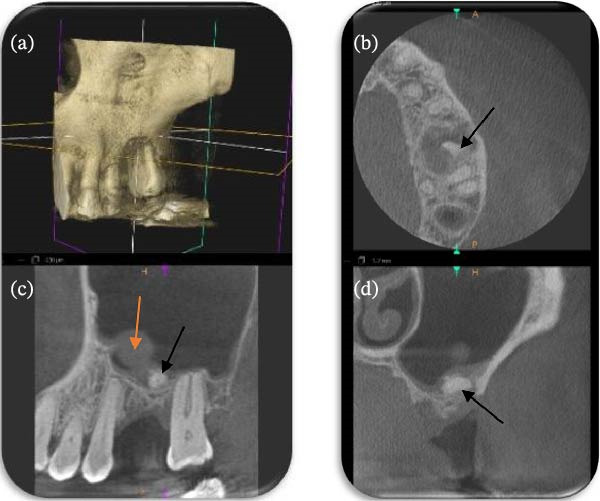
(a–d) The distobuccal root fragment embedded within thickened Schneiderian membrane in the axial, sagittal and coronal CBCT sections (black arrows). A maxillary retention cyst is also visible in the inferior wall of the maxillary sinus (red arrow).
